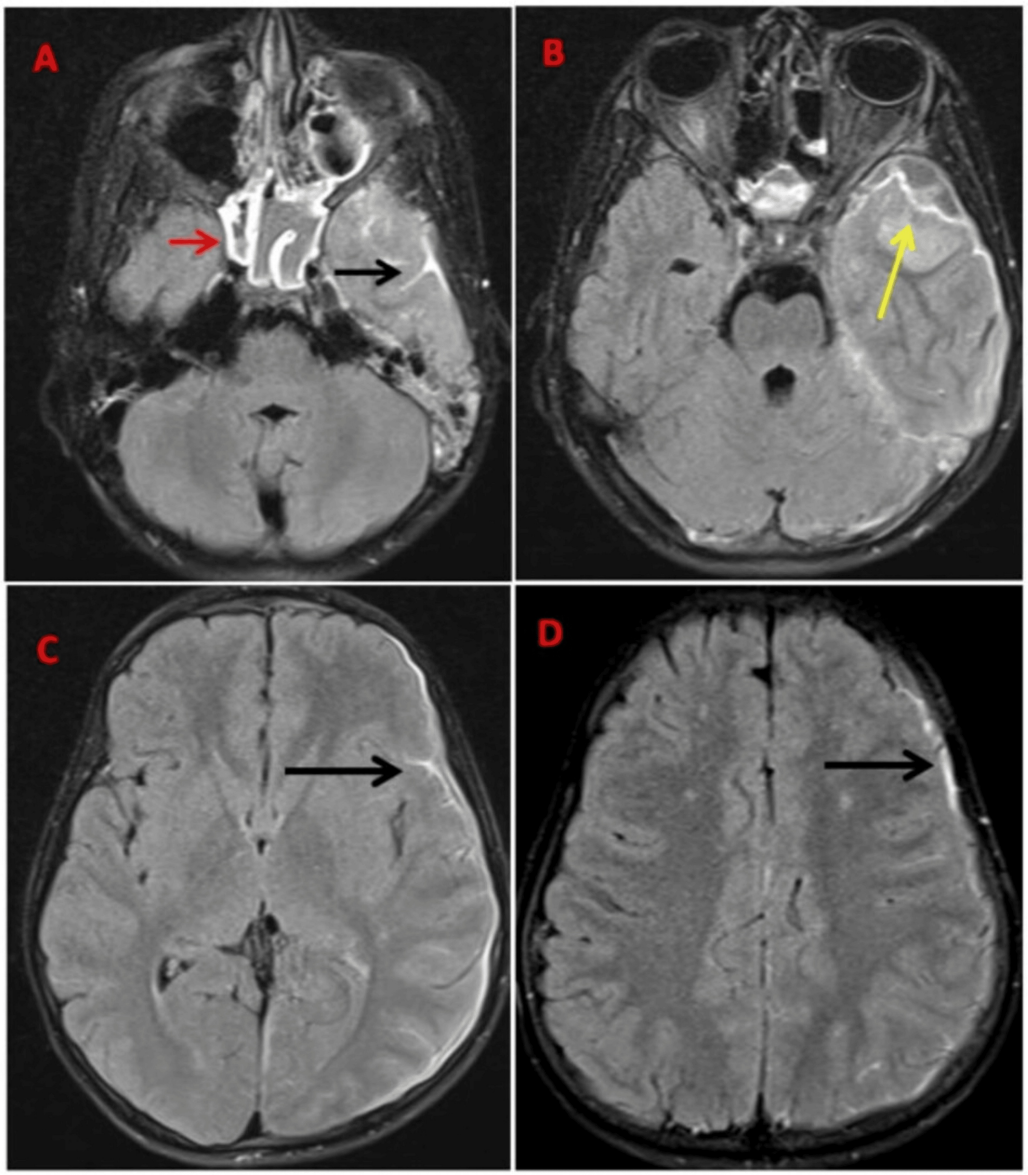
(A-D) Axial contrast FLAIR showing sphenoid sinusitis with mucosal enhancement (marked by red arrow), subdural empyema in the left fronto-temporo-parietal region (marked by yellow arrow), and pachymeningeal and leptomeningeal enhancement in the left temporo-parietal region (marked by black arrow). FLAIR: fluid-attenuated inversion recovery.
